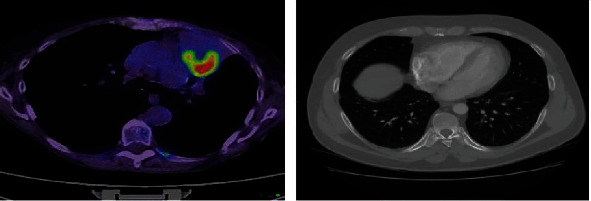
(a) F-FDG PET image; (b) LIDC-IDRI DICOM lung image.
